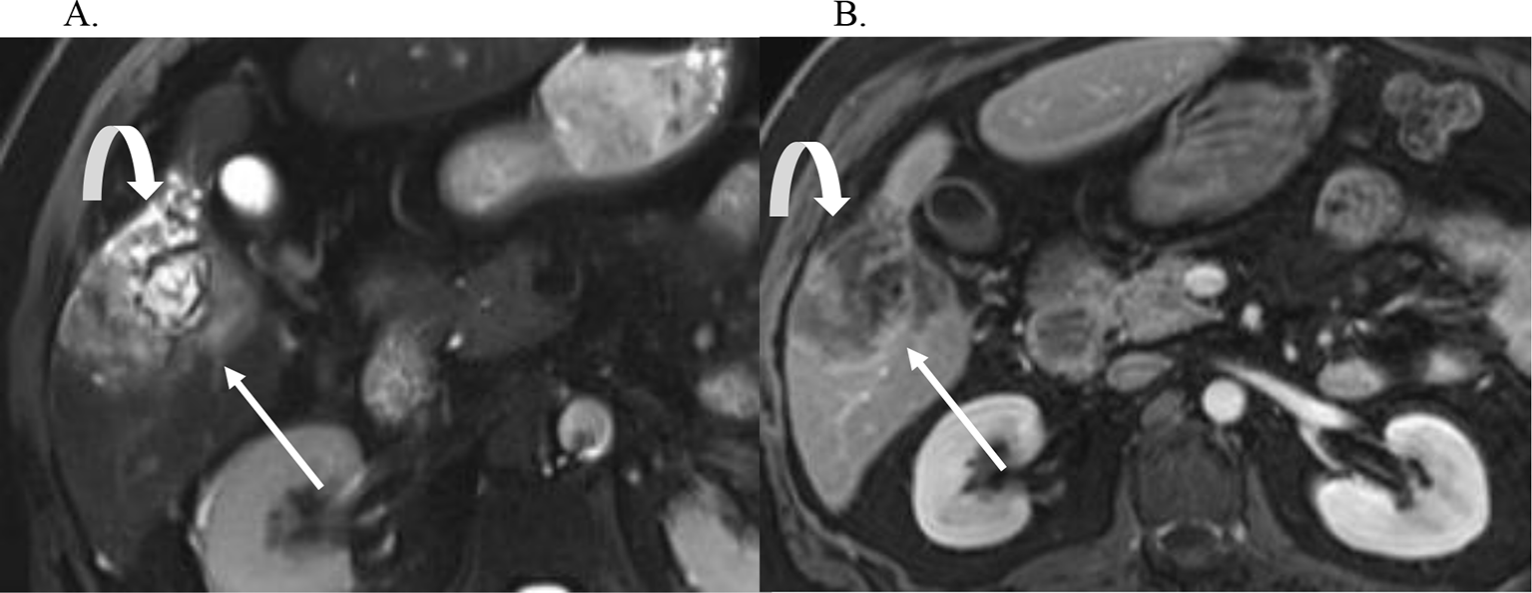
Small Duct Cholangiocarcinoma. Axial T2-weighted fat saturated image (A) and post-contrast image (B) demonstrate a T2 mildly bright mass in segment 5 of the liver (arrow) with rim enhancement and delayed central enhancement (arrow, B), consistent with a mass forming small duct CCA with associated capsular retraction (curved arrow)
